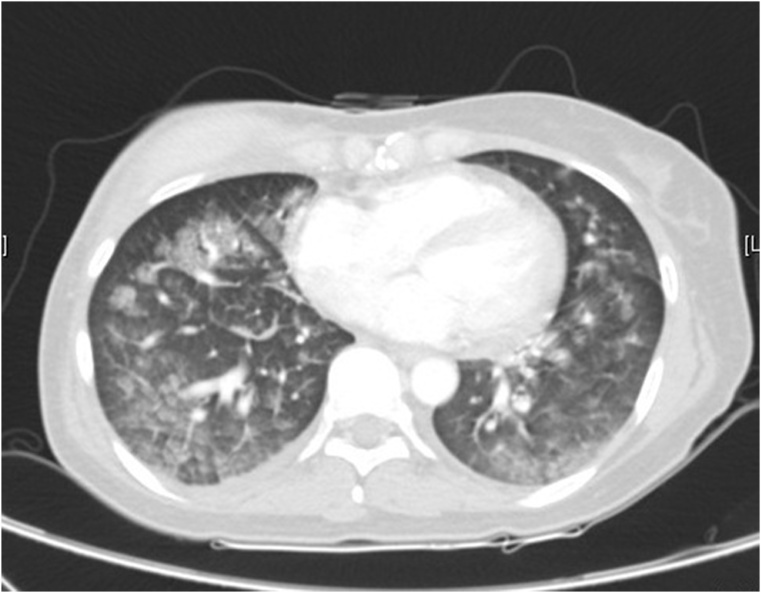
Chest CT on post-operative Day 21. Ground-glass opacification and interlobular septal thickening, and mild pleural effusion in both lungs.
